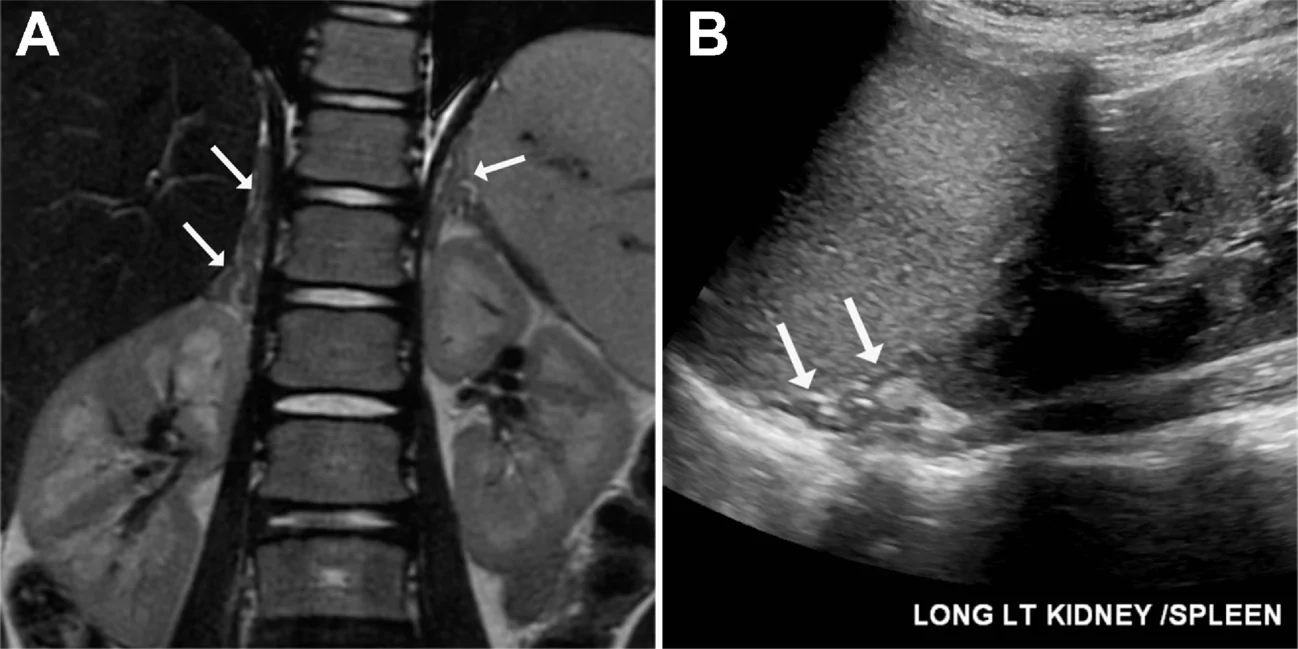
Congenital adrenal hyperplasia due to 21-hydroxylase deficiency in a 5-year-old female presenting with pubic hair at birth and later clitoromegaly. a Coronal T2-weighted abdominal MRI focused on the adrenal glands shows abnormally elongated and cerebriform morphology of bilateral adrenal glands (*arrows*). b Retroperitoneal ultrasound longitudinal view of left adrenal gland in same patient shows irregular morphology of the adrenal gland with stippled echogenicity (*arrows*)
